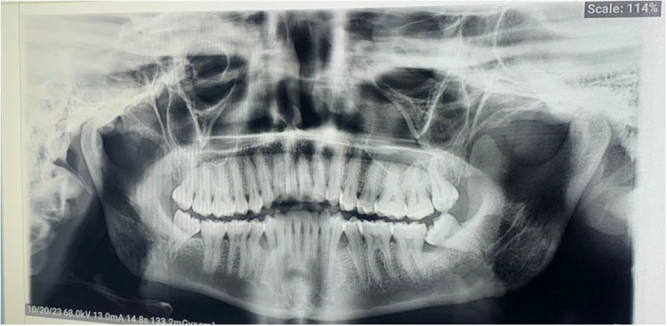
Postoperative orthopantomogram.
